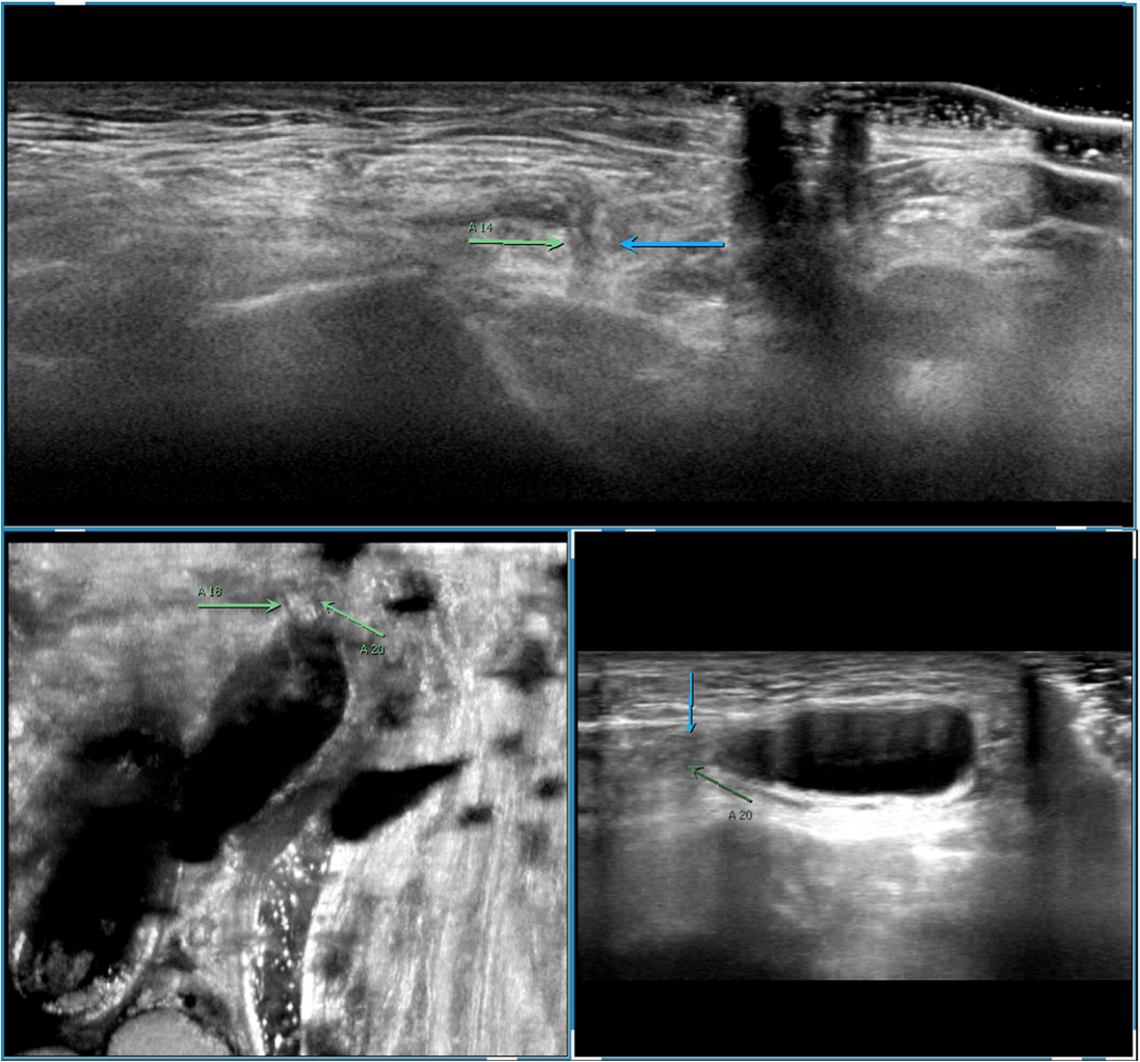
The cyst of spermatic cord showed in the ultrasonography. Arrows referred to the left spermatic cord
